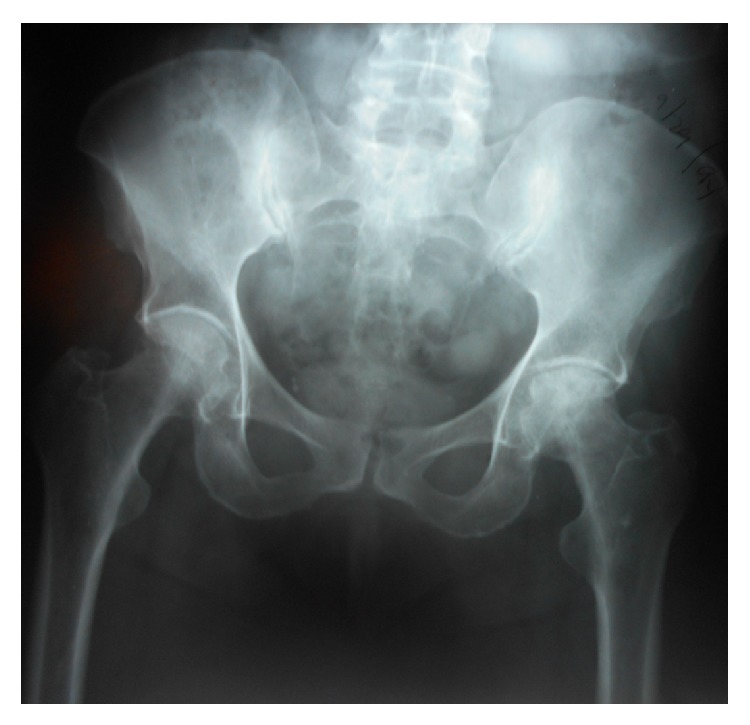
Anterior-posterior hip X-rays in patient number 4 at preanticoagulation study entry. The right hip is Ficat stage II and the left hip is Ficat stage III.
